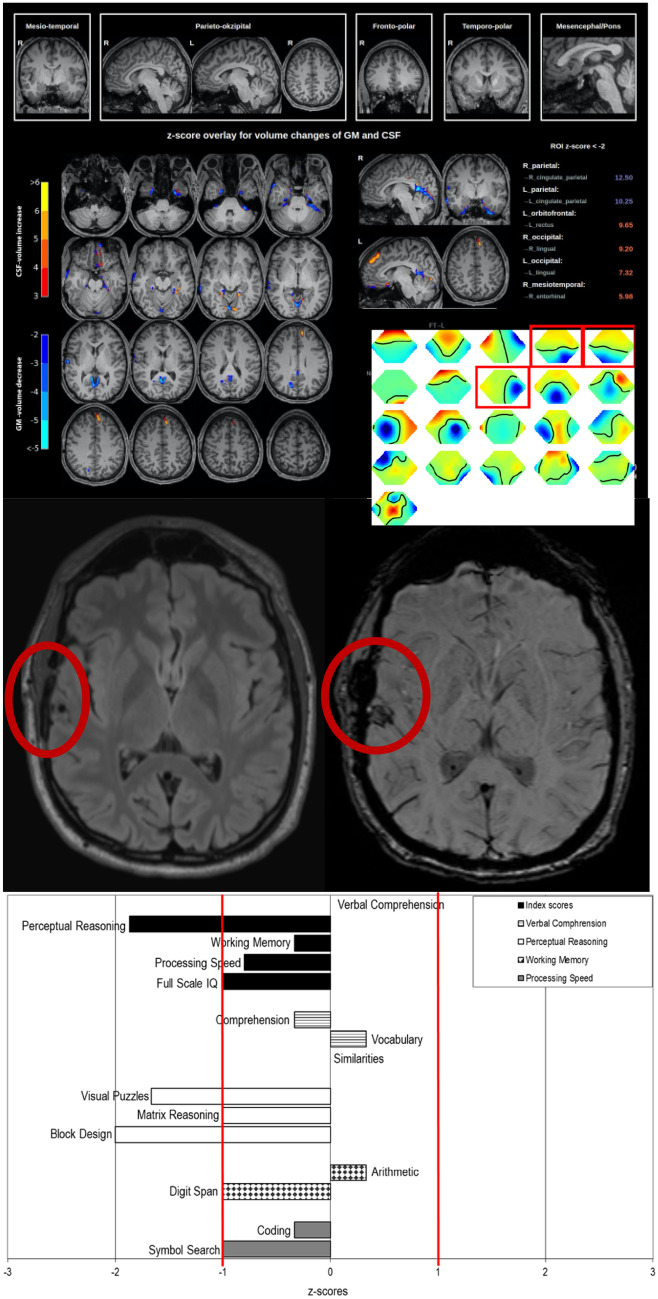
A combined volume- and region-based analysis method (above) using the MPRAGE magnetic resonance imaging (MRI) sequences identified grey matter (GM) volume loss in the right (z-score: 12.5) and left (z-score: 10.25) parietal cortex. Atrophic changes with increased cerebrospinal fluid (CSF) volume were identified in the left orbitofrontal cortex (z-score: 9.65), right (z-score: 9.2) and left (z-score: 7.32) occipital cortex, and the right mesiotemporal cortex (z-score: 5.98). FLAIR and SWI sequences (central) showed a small brain parenchymal defect right temporal with hemosiderin deposits after cavernomectomy. The inset shows the electroencephalography independent component analysis maps. The components showing intermittent rhythmic delta activity (right and left occipital, right temporal) are framed. The Wechsler Adult Intelligence Scale—Fourth Edition yielded an overall intelligence quotient of 85 (processing speed score of 88, working memory score of 95, perceptual/logical reasoning score of 72, and verbal comprehension score of 100; bottom). *FLAIR* fluid attenuated inversion recovery, *MPRAGE* magnetization prepared rapid gradient echo, *NPH* normal pressure hydrocephalus, *SWI* susceptibility-weighted imaging
